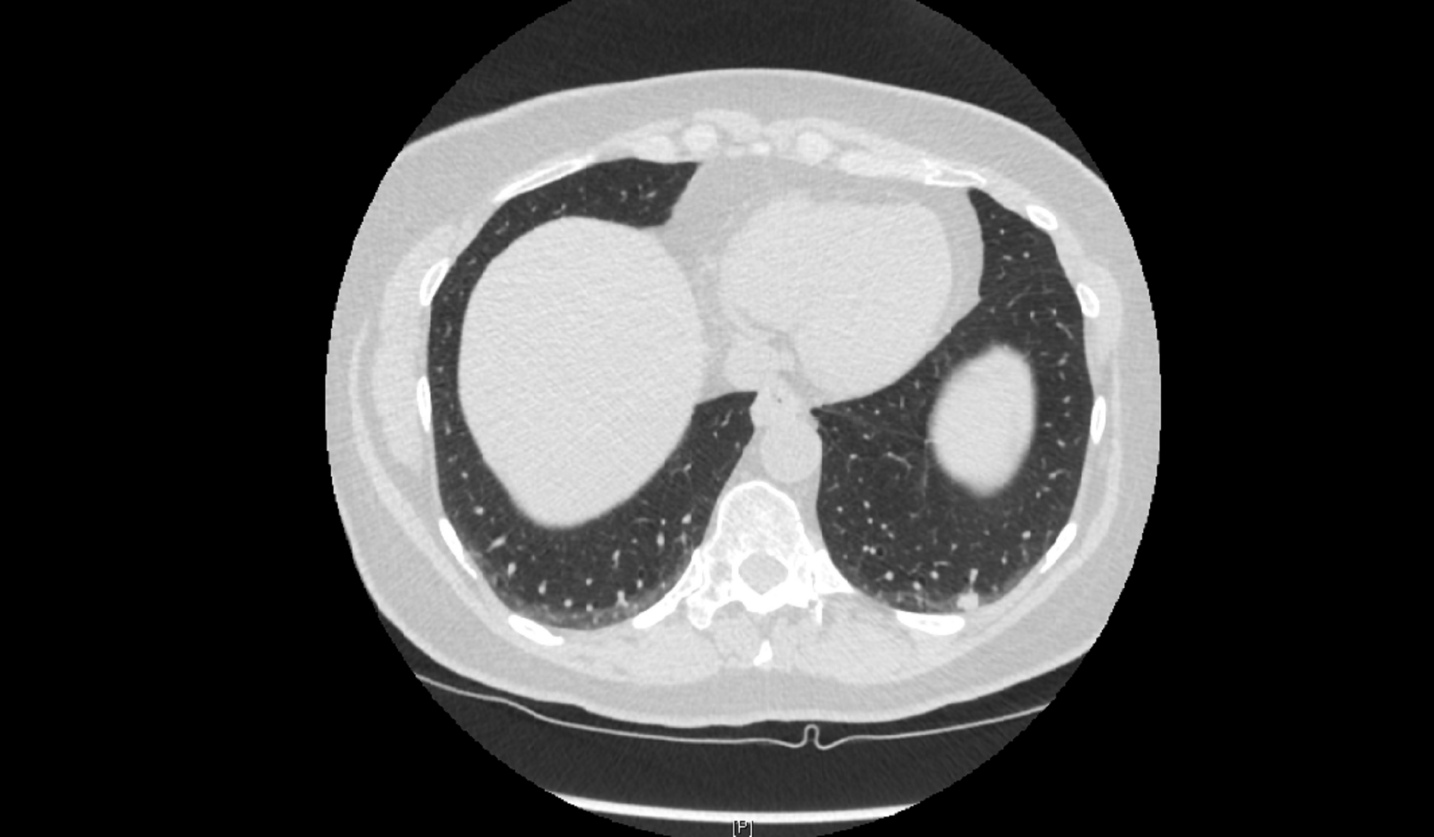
CT scan of the chest in November 2011 New 9 mm solid nodule in the posterior left lower lobe is seen.
